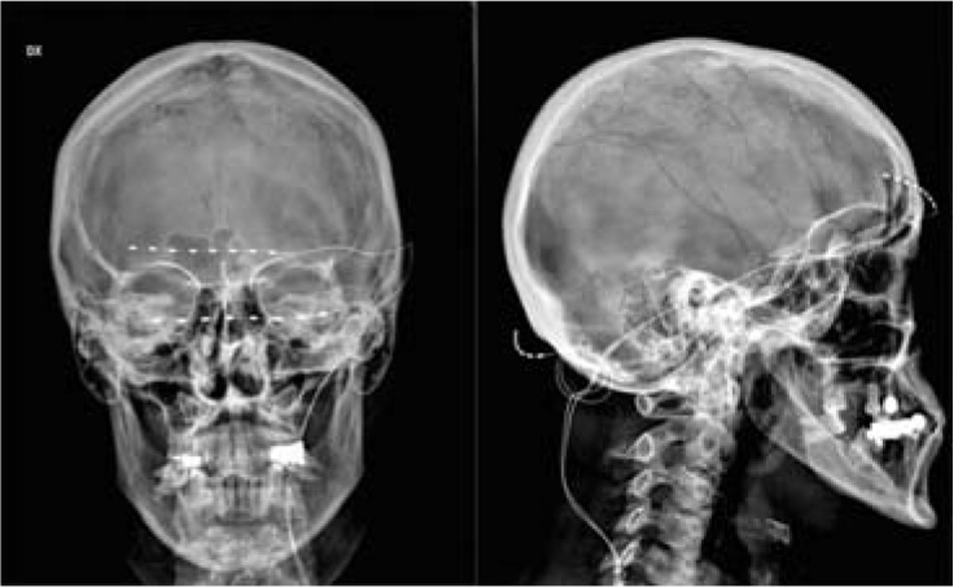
Radiographic image revealing multiple - ONS plus SNO - implant in rCCH patient.
